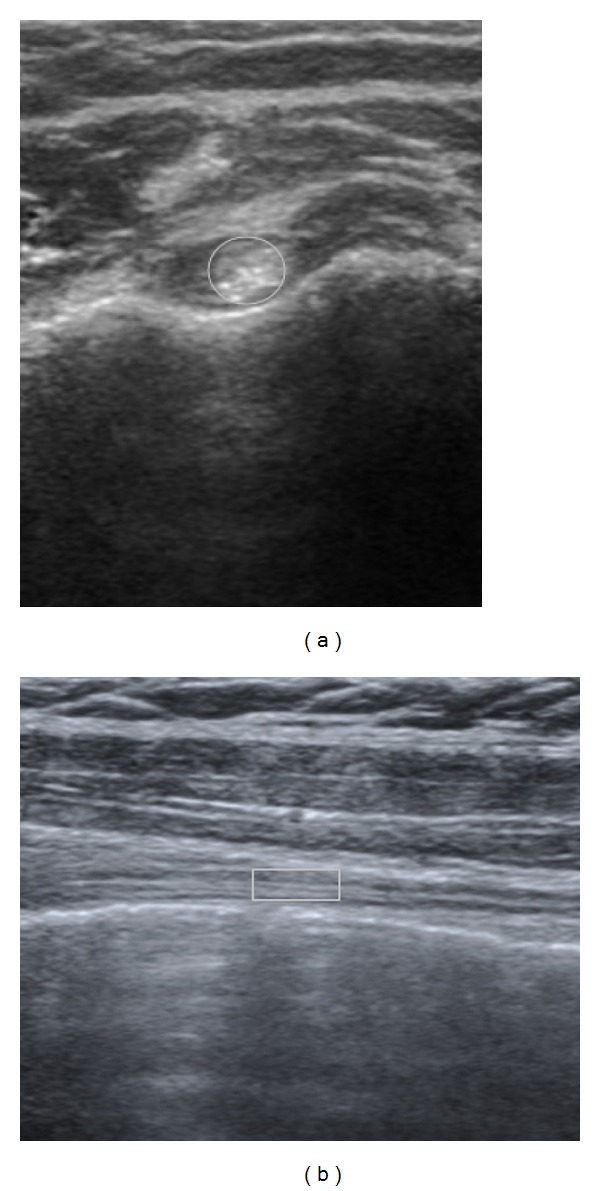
Biceps ultrasound. (a) Transverse view of the biceps tendon showing hypoechogenicity surrounding the bicipital tendon. The ROI was defined in a circular area, which covers the biceps tendon sheath. (b) The hypoechogenicity pattern among the biceps tendon is shown in this longitudinal view. The ROI was selected to be in the part of the biceps tendon with the most significant fluid accumulation, and the boarder part of this square area is defined as being located on the biceps tendon sheath. The grayscale values of the ROI were directly calculated by using the PACS system.
